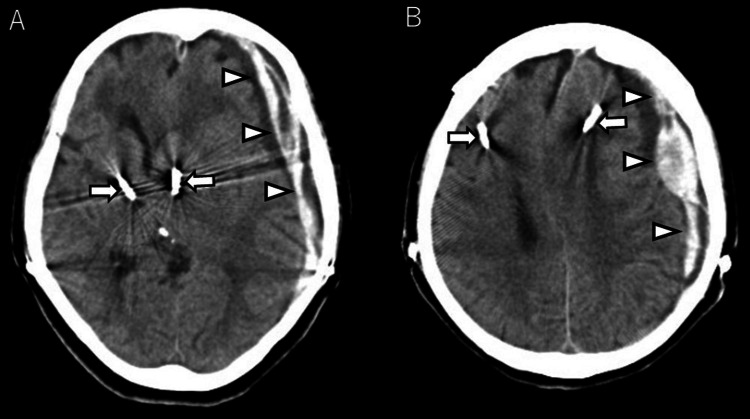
Initial non-contrast head computed tomography (CT) (A, B) Initial non-contrast head computed tomography showing a thick acute subdural hematoma (arrowhead) and midline shift and significant displacement of the deep brain stimulation electrodes (arrow) with the overall brain shift.
